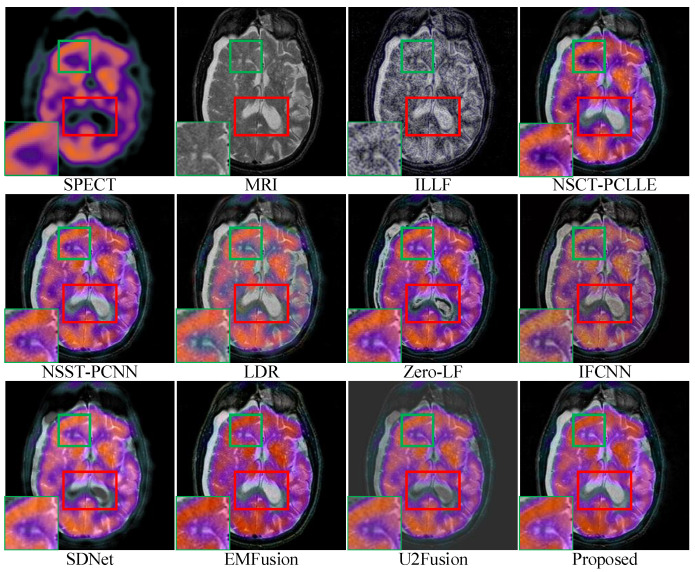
Comparison of performances of various methods on the single-photon emission computed tomography (SPECT)-MRI images. For a clear comparison, we select two same regions (i.e., the green and red boxes) in each image, and the green boxes are zoomed in the bottom left corner.
